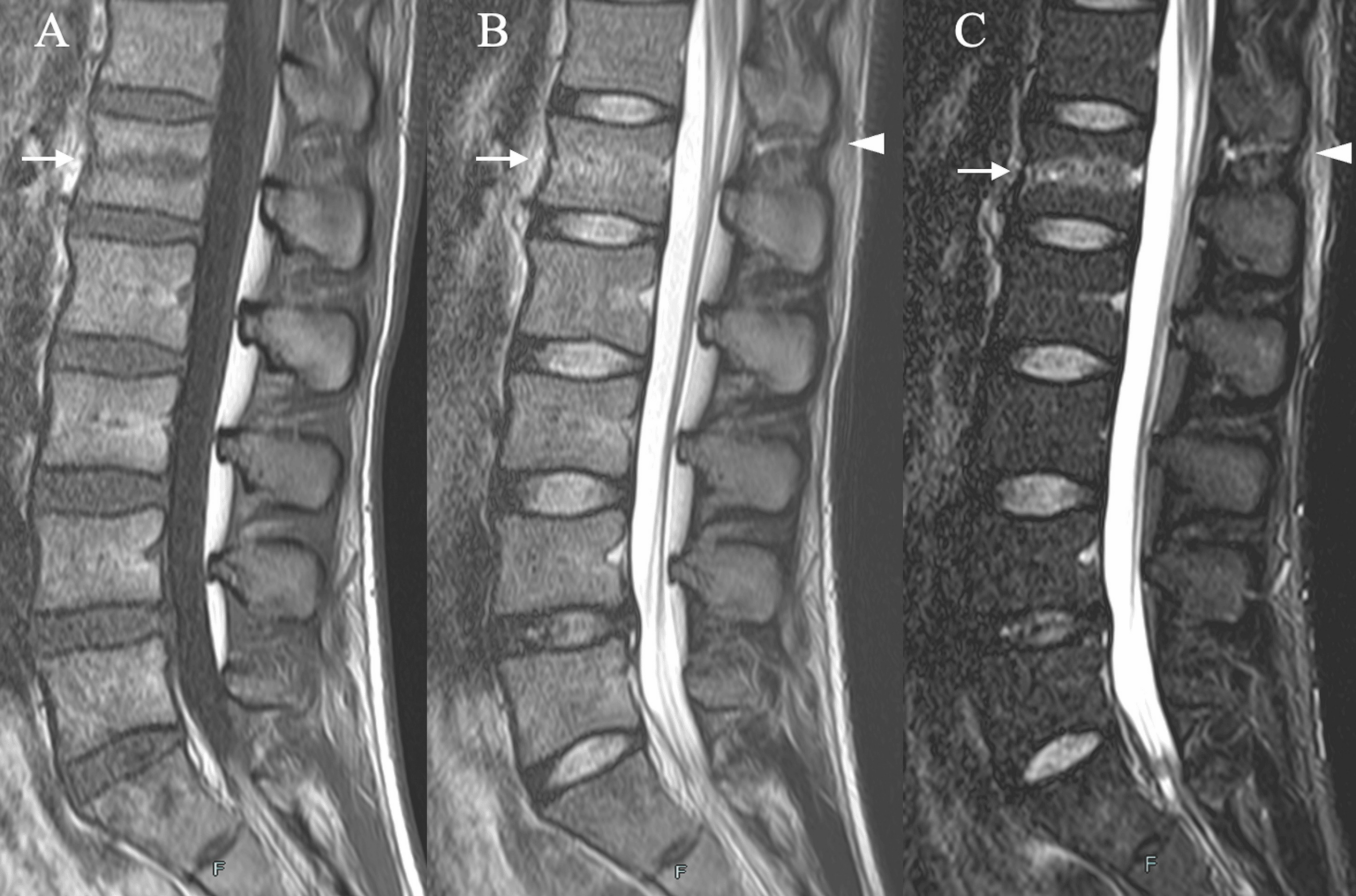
MRI findings (A) T1-weighted (B) T2-weighted (C) STIR MRI sagittal view of the spine showing a fracture of the L1 vertebra (white arrows) and disruption of the supraspinous and interspinous ligaments at the T12–L1 level (arrowhead). MRI: magnetic resonance imaging, STIR: short tau inversion recovery
